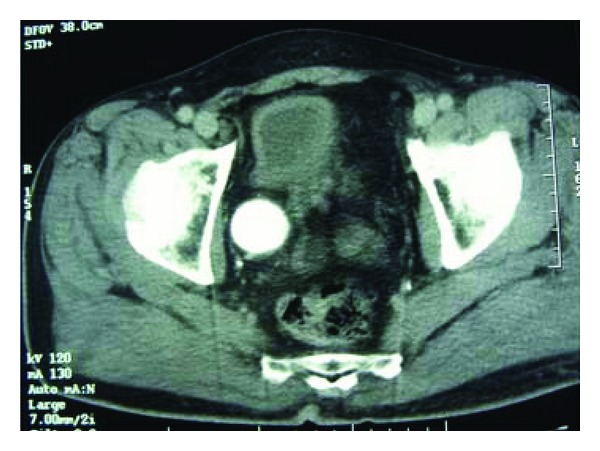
CT of the same patient showing pelvic ureteral stone.
